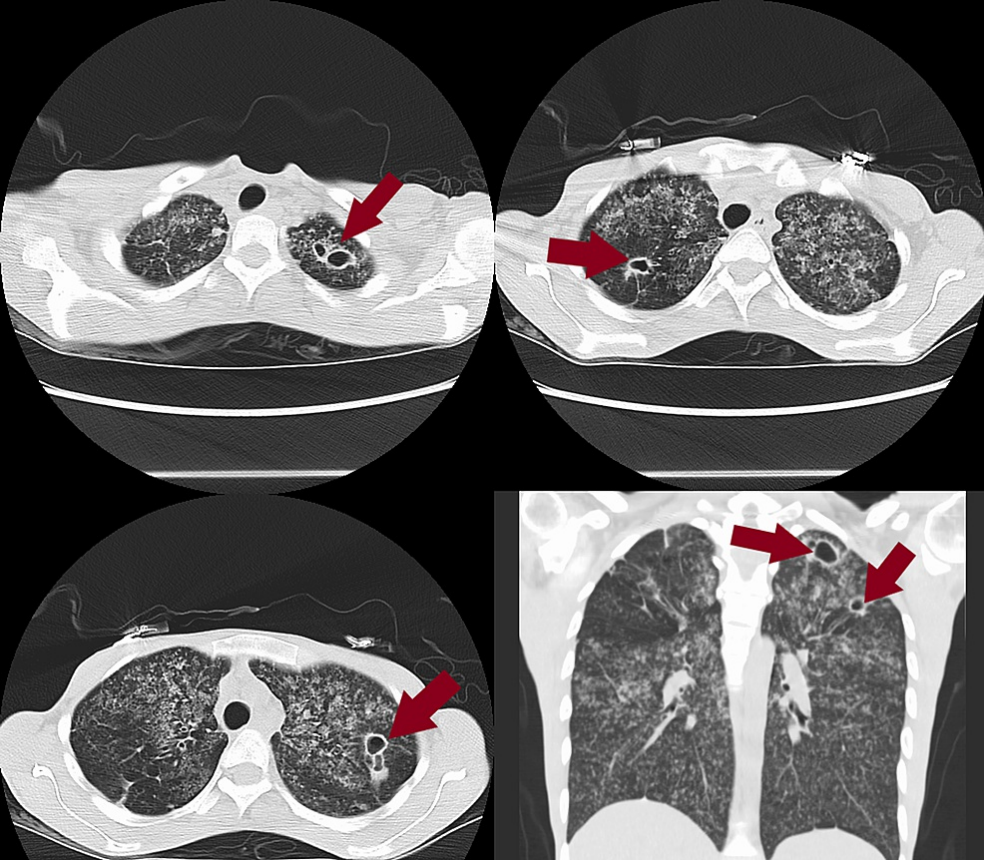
CT chest. Diffuse bilateral lung disease with areas of cavitation (red arrows). CT, computed tomography.
